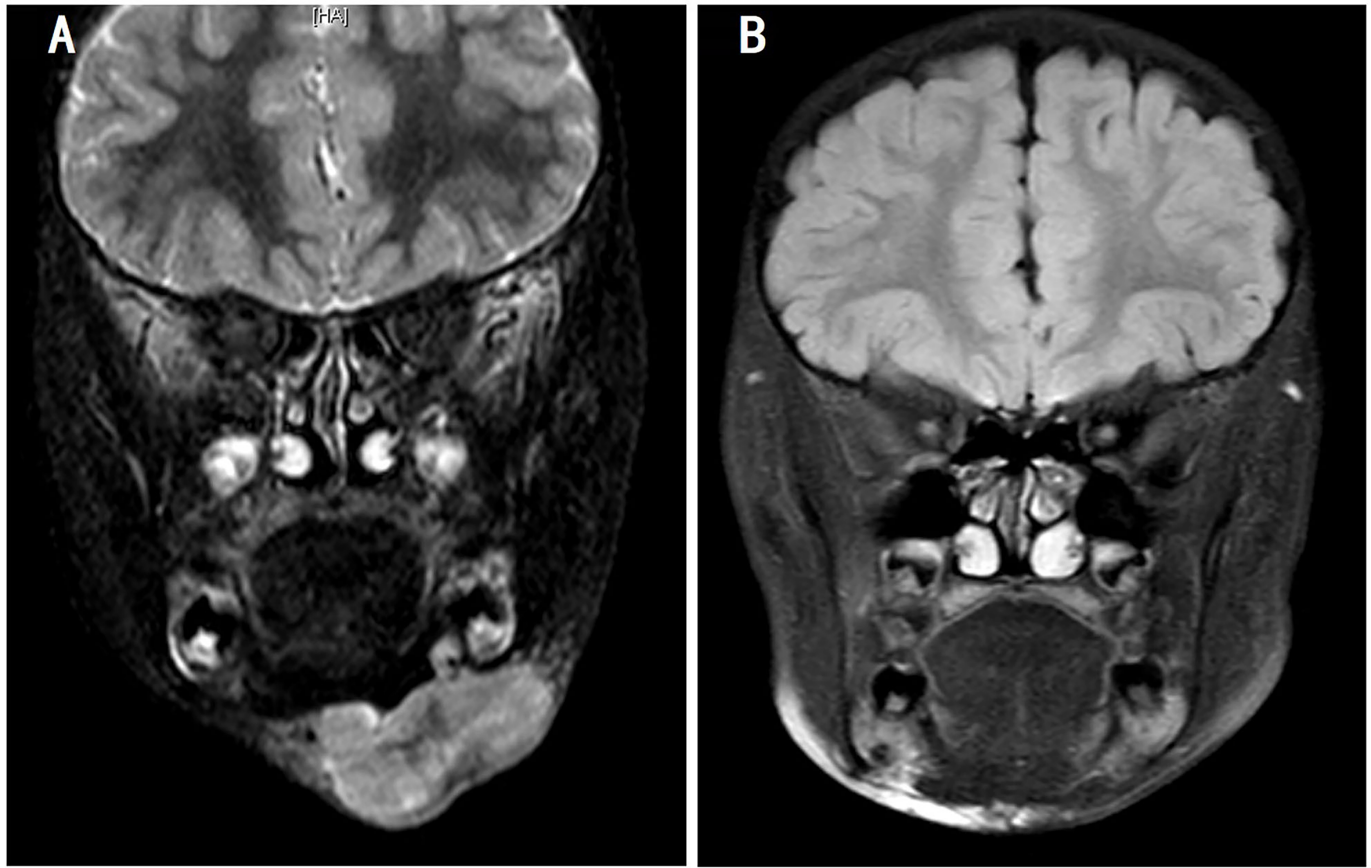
Magnetic resonance imaging of the changes in patient 2. (A) Before ATO treatment and (B) 3 weeks after three courses of treatment with the ATO-combined chemotherapy (five courses in total). ATO, arsenic trioxide.
